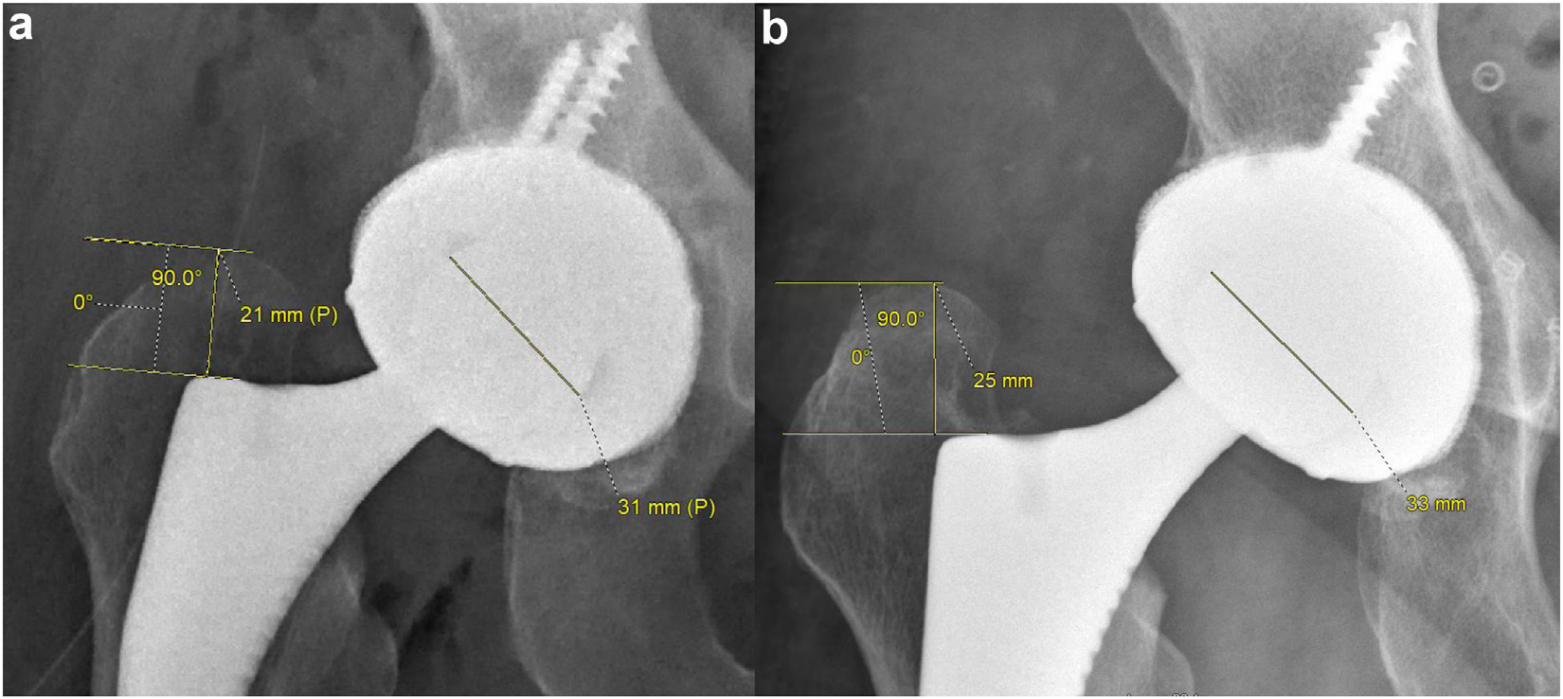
Example of subsidence measurement comparing immediate post-operative radiographs (a) with those taken at 6 weeks postoperatively (b). Known femoral head size was used for magnification correction.
